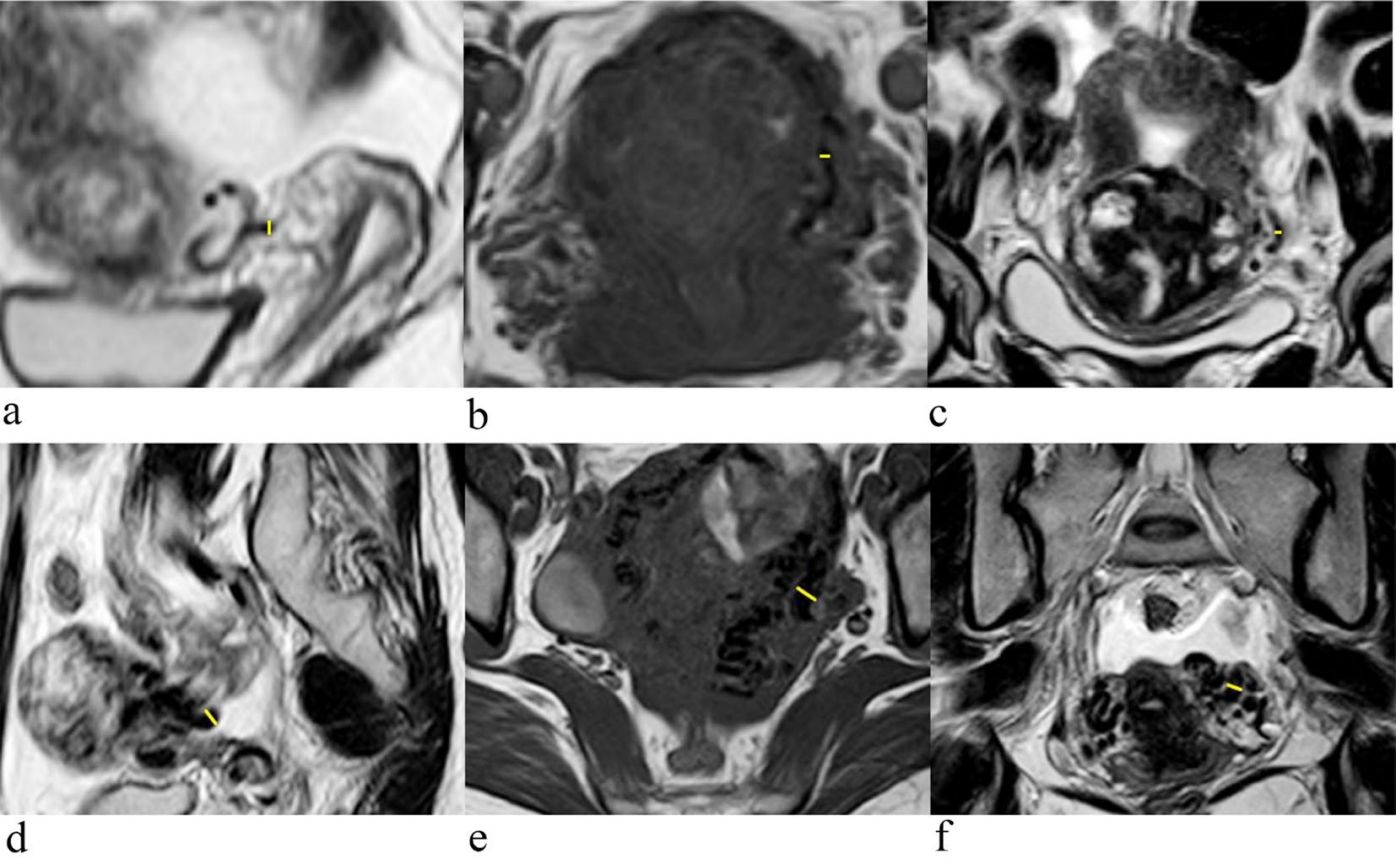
Images obtained of a 30-year-old woman with RPOC without uterine AVMs. The fv-D measurements in the parametrium on MRI. (a) Sagittal T2WI (fv-D = 2.3 mm). (b) Axial T1WI (fv-D = 2.0 mm). (c) Coronal T2WI (fv-D = 2.7 mm). The mean value is 2.3 mm. Images obtained of a 32-year-old woman with RPOC coexisting with a uterine AVM. The fv-D measurements in the parametrium on MRI. (d) Sagittal T2WI (fv-D = 5.5 mm). (e) Axial T1WI (fv-D = 5.4 mm). (f) Coronal T2WI (fv-D = 5.6 mm). The mean value is 5.5 mm.
